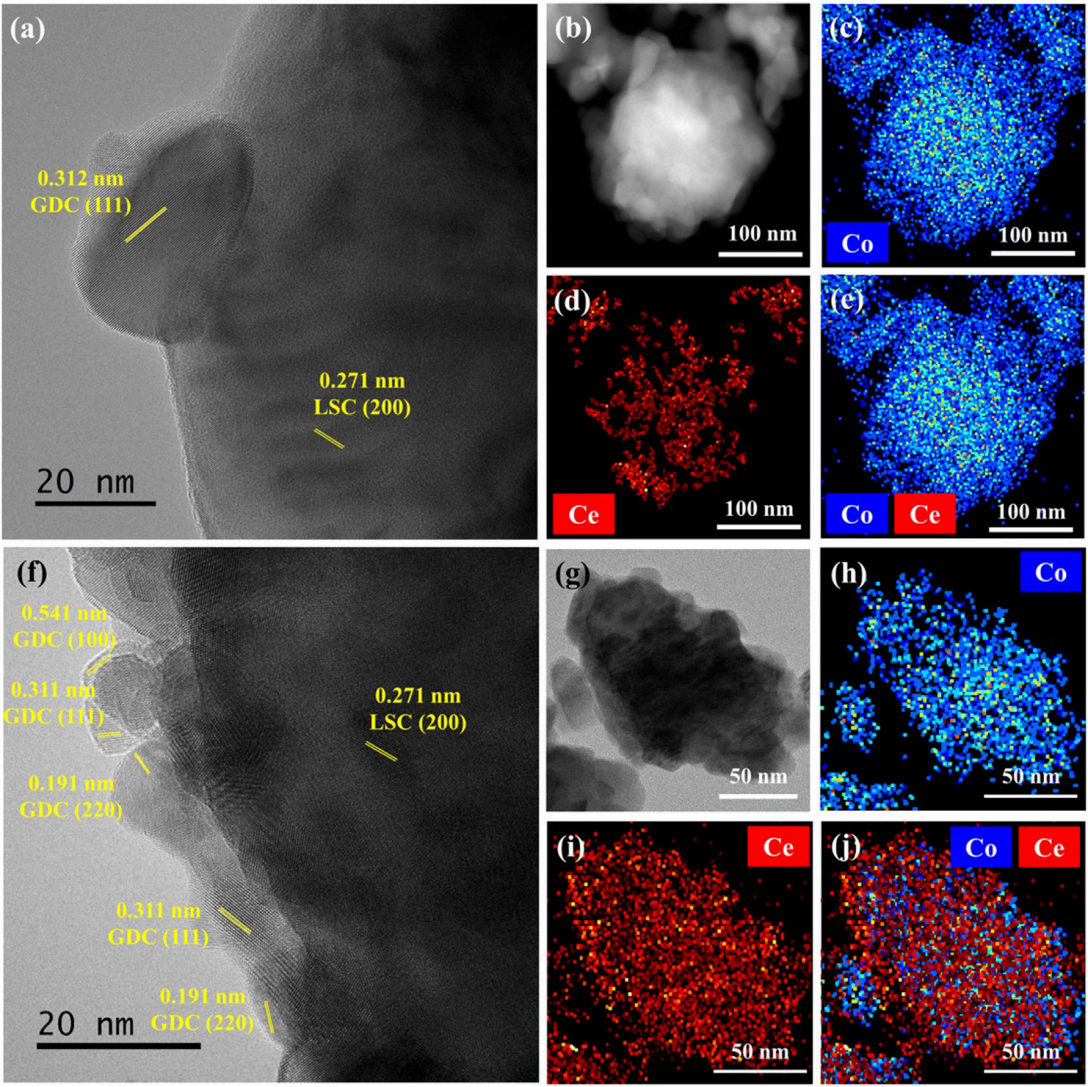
HR-TEM images of LSC-GDC powders prepared using (A) conventional ESD and (F) SGC-assisted ESD. TEM-Energy dispersive X-ray dispersive analysis (EDAX) elemental mapping of Co. and Ce for LSC-GDC powders using (B–E) conventional ESD and (G–J) SGC-assisted ESD.
